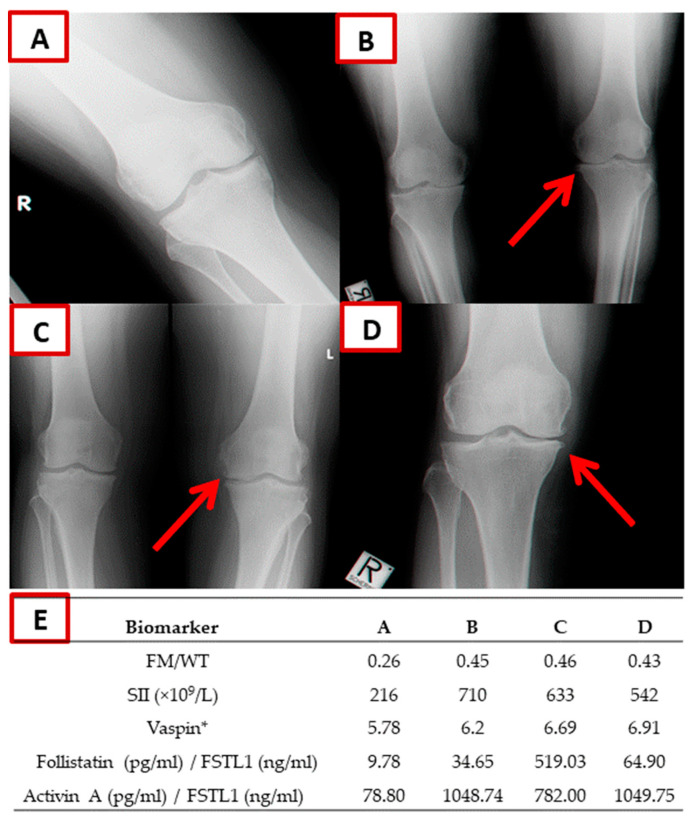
Examples of X-ray knee images. The figure shows two typical incidents of degenerative osteoarthritis of the knee joint in three individuals with KOA compared with a healthy individual. (A) A 55-year-old woman with a normal knee, and (B) a 53-year-old woman with severe KOA, accompanied by joint space narrowing in both legs at the medial aspect of the knee joint compared to its lateral aspect. Also, osteophytes are observed at the medial aspect of the knee joint (red arrow). In addition, sclerosis is observed in both knees. (C) A 51-year-old woman with severe KOA: joint space narrowing with osteophytosis in the left leg (red arrow); (D) a 55-year-old man with severe KOA in the left leg, accompanied by joint space narrowing and osteophytes (red arrow); and (E) the blood biomarker levels of these participants are presented in the table. * Plasma levels of the vaspin are presented after log-transformation to approximate normality prior to analysis.
